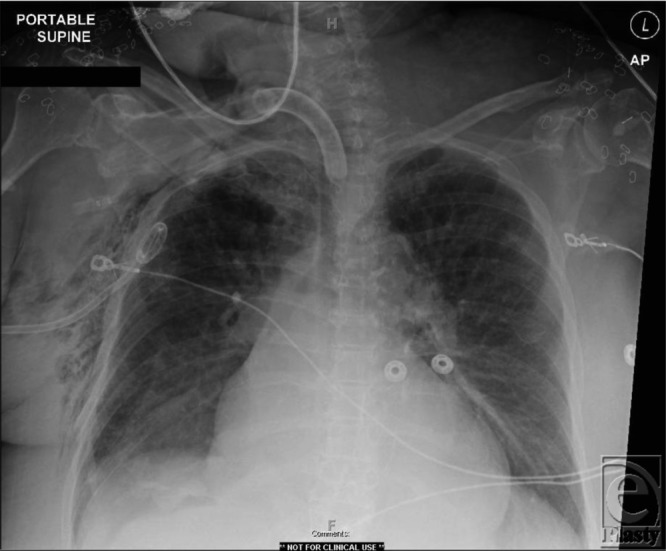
Chest radiograph following decompression of right pneumothorax.
